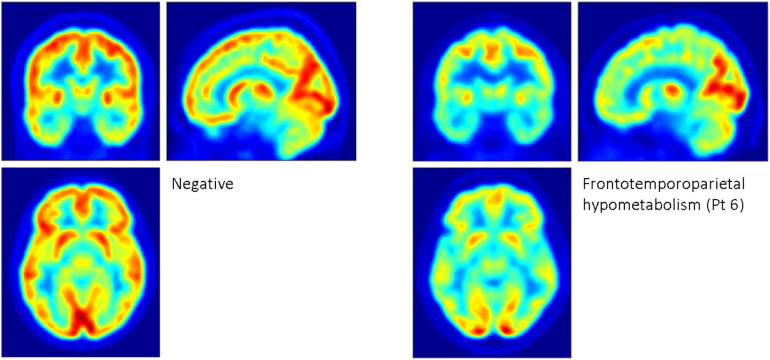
Examples of patterns of glucose metabolism revealed by [^18^F]FDG PET. The panel on the left depicts the mean of two patients who had scans that were reported as normal. The panel on the right depicts marked, widespread frontotemporoparietal hypometabolism sparing the occipital cortex, seen in patient 6, a 40-year-old male with full-scale IQ of 90 who ceased adherence to the low phenylalanine diet before he reached 10 years of age. His most recent blood spot phenylalanine measurement prior to the scan was 1200 μmol/ml. Based on 25 phenylalanine and 22 tyrosine measurements, his mean ± standard deviation historical phenylalanine was 877.2 ± 183.6 μmol/ml, and his Phe:Tyr ratio was 89.2 ± 52.6.
